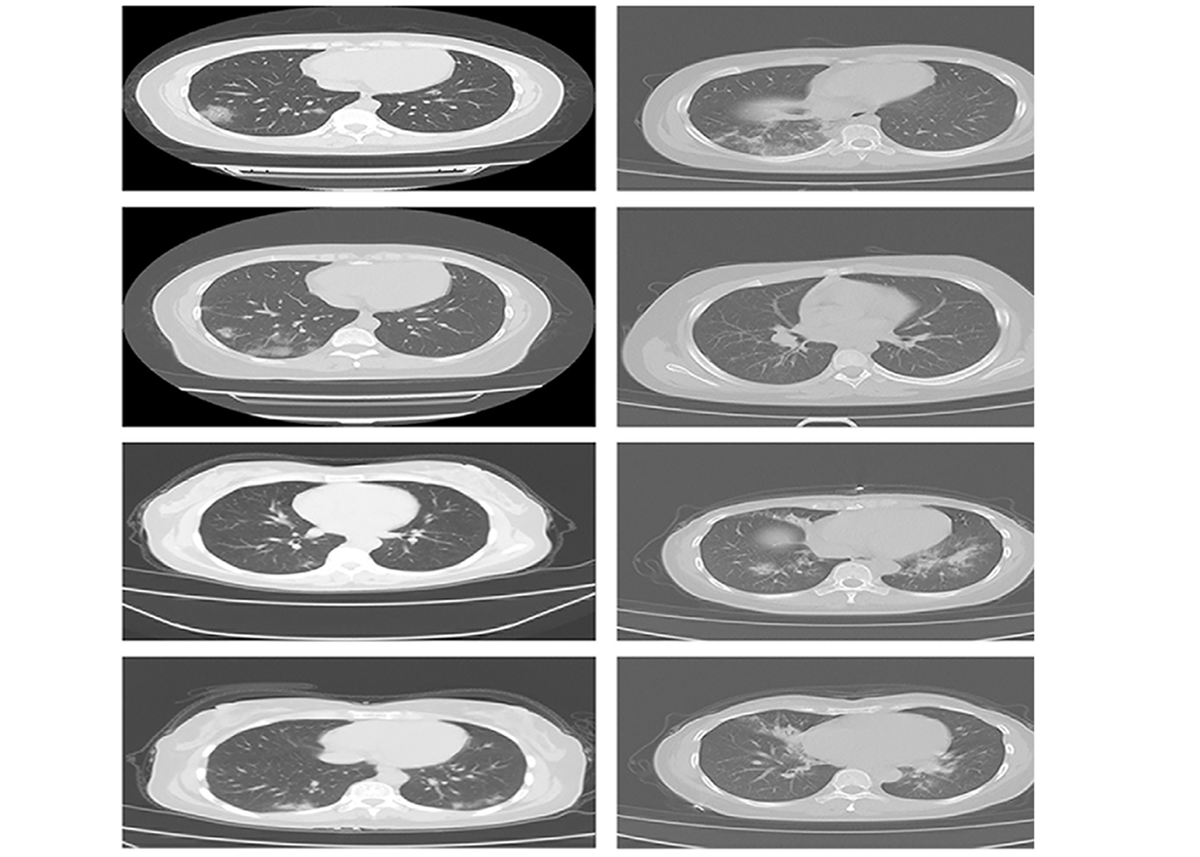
The images in the left panel are CT scans of four randomly selected confirmed COVID-19–positive patients. There are multiple patchy lesions, and the grinding glass shadows are distributed in the peripheral pulmonary field. The images in the right panel are CT scans of four randomly selected suspected patients who were confirmed to be COVID-19 negative. Their lesions are large, strip-like, and have uneven density. Pleural thickening near affected lung segments is also seen.
